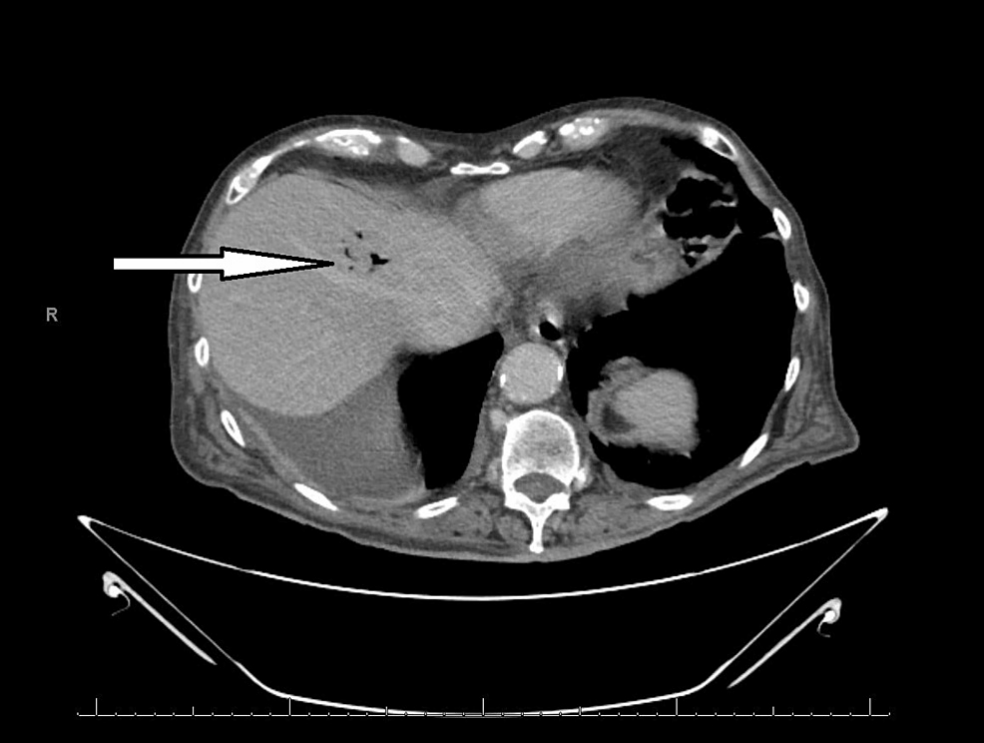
Axial plane CT abdomen and pelvis obtained shortly after admission showing branching air in the left hepatic lobe (arrow) which could represent pneumobilia.
